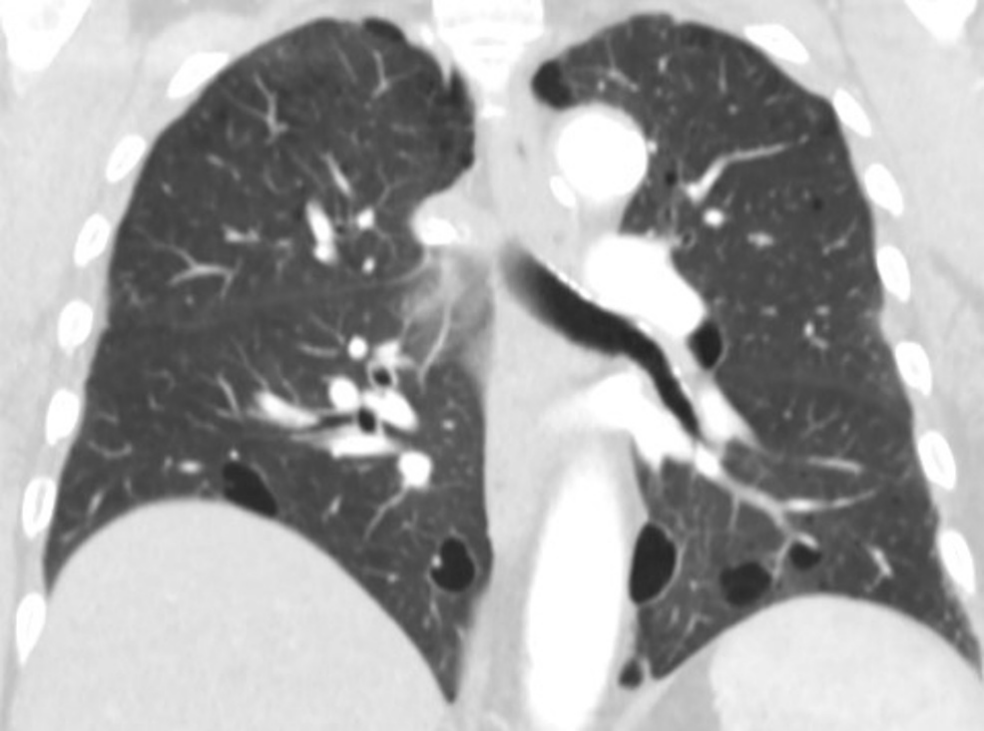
Enhanced computed tomography (CT) scan of the chest (coronal view) demonstrating the presence of bilateral thin wall elliptical-shaped cysts, in the middle and lower lobes of both lungs, with no other ancillary findings.
